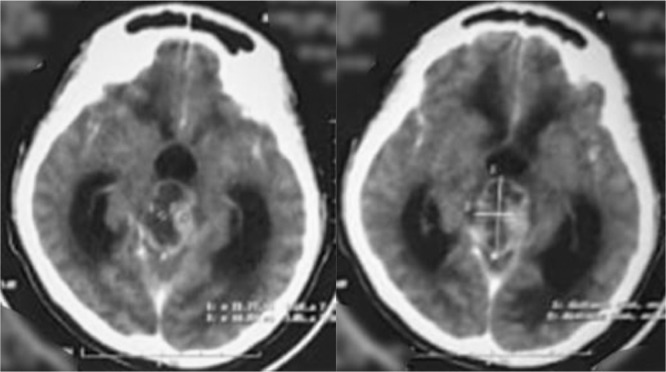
CT Scan of head with contrast (axial section).
